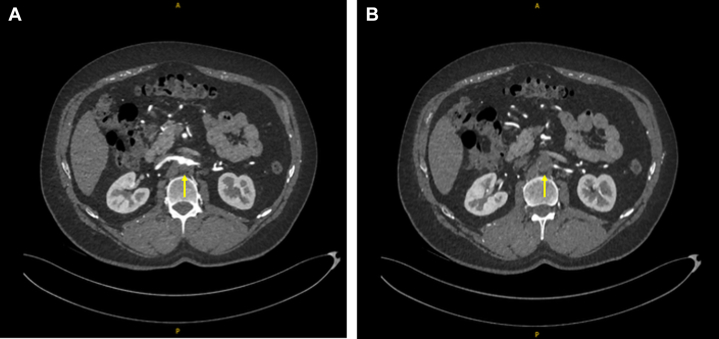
Axial Images From a Computed Tomography Aortogram With Arterial Contrast Demonstrating Complete Infrarenal Aortic Occlusion (Yellow Arrows) (A) Patent proximal right renal artery is shown just above the level of occlusion. (B) The start of the occluded aortic segment is shown.
